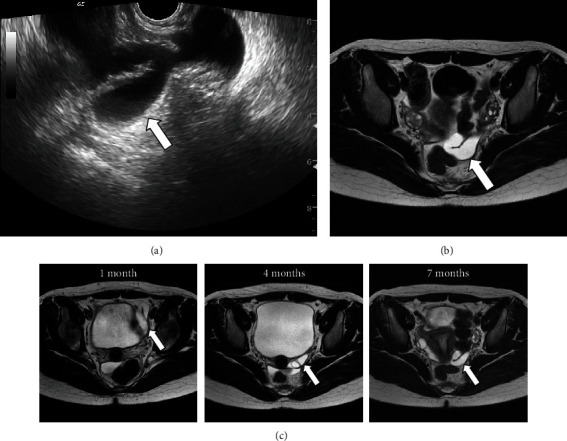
(a) Transrectal ultrasound appearance of a cystic mass (arrow). (b) Axial T2-weighted magnetic resonance imaging (MRI) at the first hospitalization revealing a normal ovary and a hydrosalpinx (arrow). (c) The hydrosalpinx shows a gradual reduction in size (arrows) on MRI.
